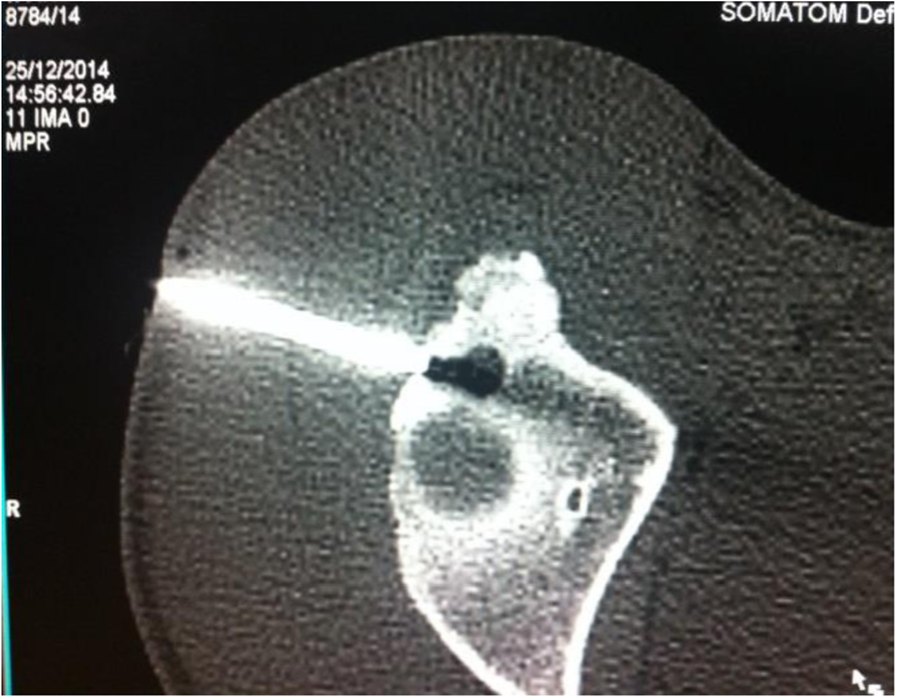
Computed tomography control immediately after resection confirmed the complete resection of osteoid osteoma
